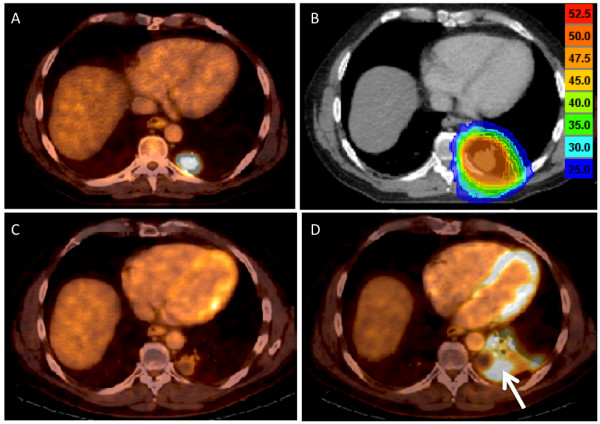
Complete metabolic response displayed in a colorectal cancer patient with a solitary lung metastasis. (A) Pre-treatment ^18 ^F-fluorodeoxyglucose (FDG)-positron emission computed tomography (PET-CT) of a metastatic colorectal cancer patient with a solitary metabolic active lungmetastasis. (B) Planning CT with superimposed radiation dose distribution. (C) Complete metabolic remission on PET-CT scan 3 months after initiation of radiotherapy. (D) PET-CT performed 6 months after completion of radiotherapy shows no evidence of progressive disease, but note the occurrence of an asymptomatic radiation induced pneumonitis around the irradiated metastasis (white arrow).
